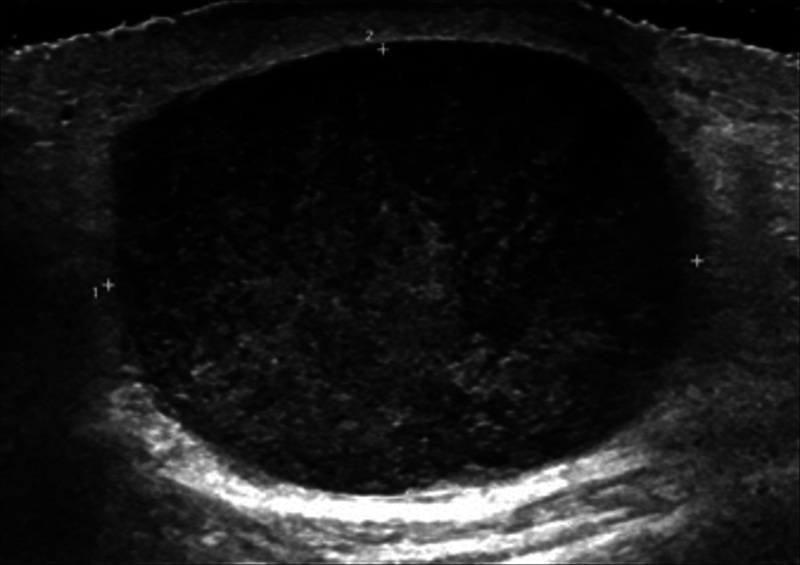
Ultrasound revealed a heterogeneously hypoechoic subcutaneous mass lesion with prominently increased vascularity and perilesional soft tissue edematous changes.
